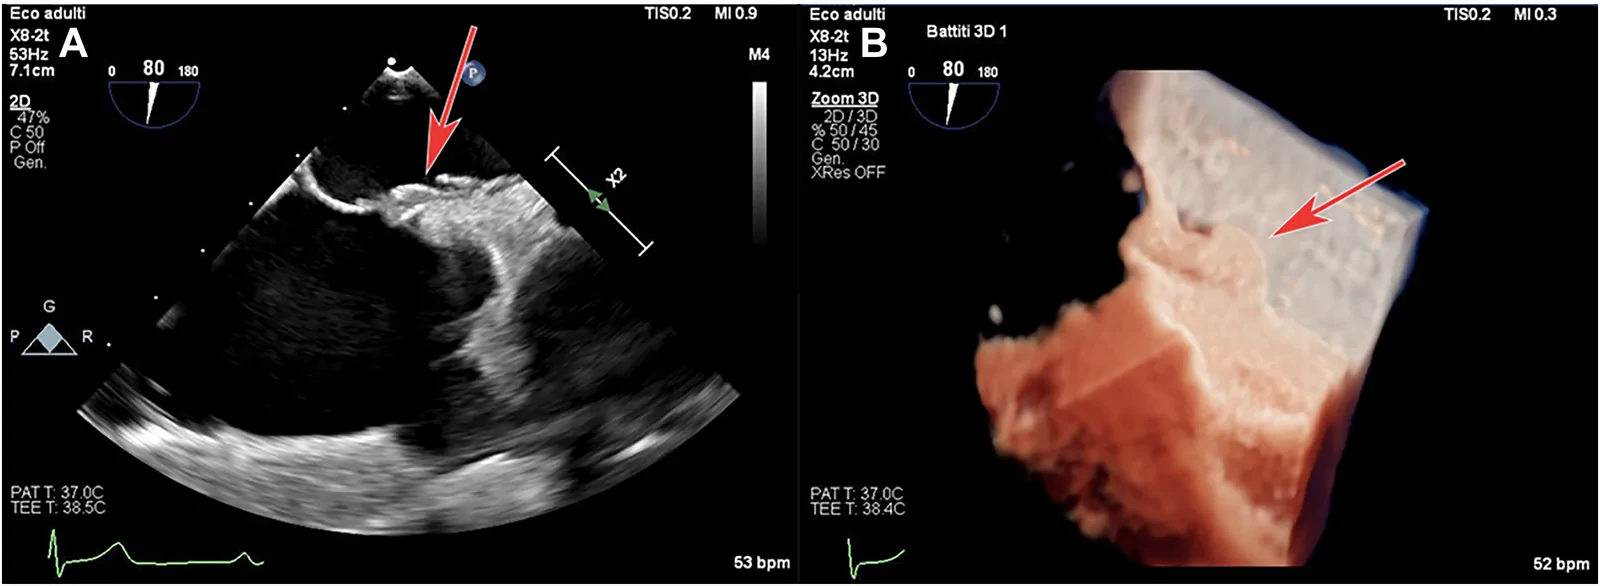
Post-Procedural Collapse and Left Atrial Inversion of the Septal Pouch The pouch (marked by red arrows), collapsed and inverted toward the left atrium on 2-dimensional transesophageal echocardiography (A) and 3-dimensional transesophageal echocardiography (B).
